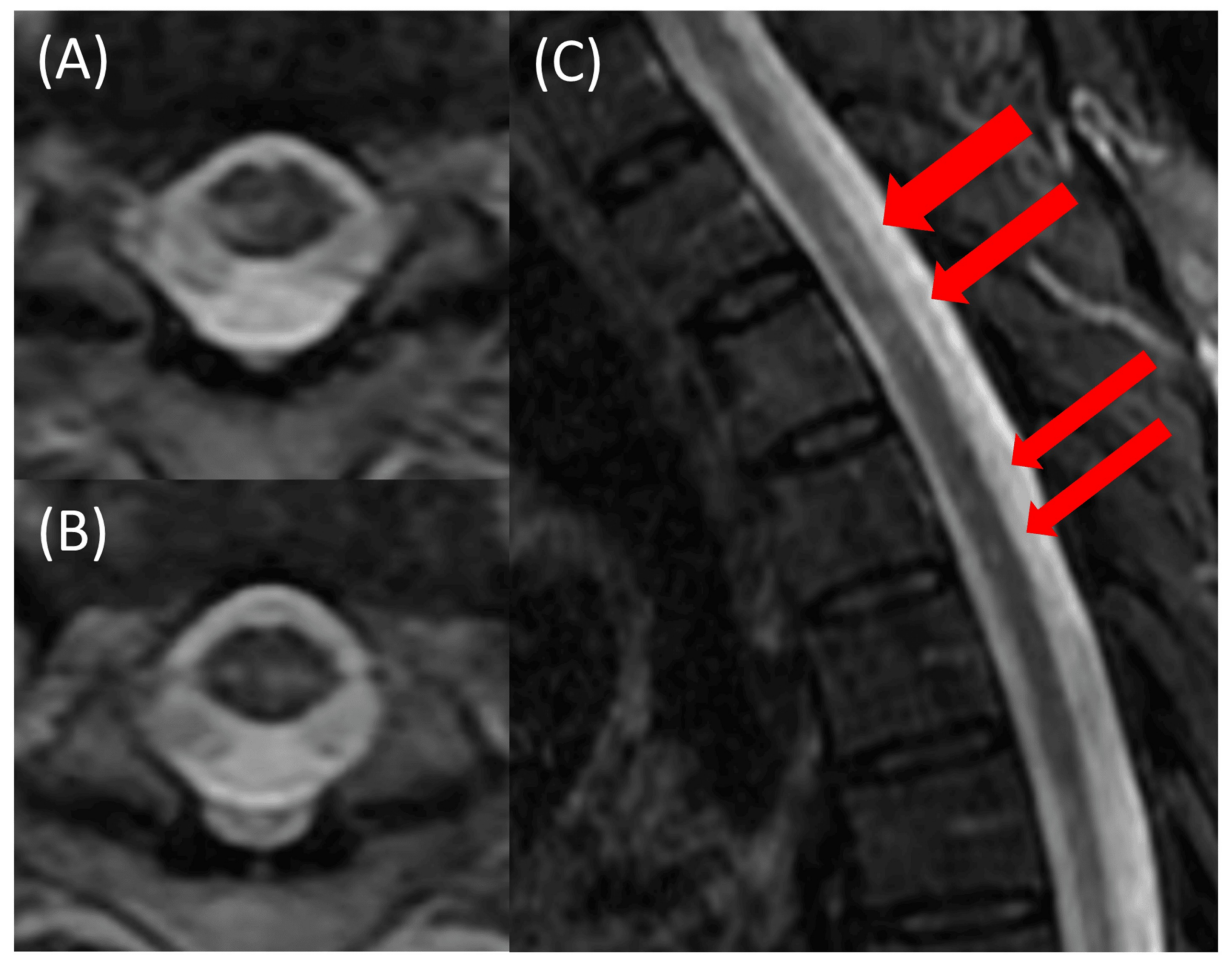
Thoracic spinal cord MRI in Patient 4 Thoracic spinal MRI showed a longitudinally extensive T2 hyperintense area with some discontinuities (A-C). The red arrows indicate the T2 hyperintense region. (A) and (B) correspond to the uppermost and lowermost slices at the locations of the arrows, respectively.
